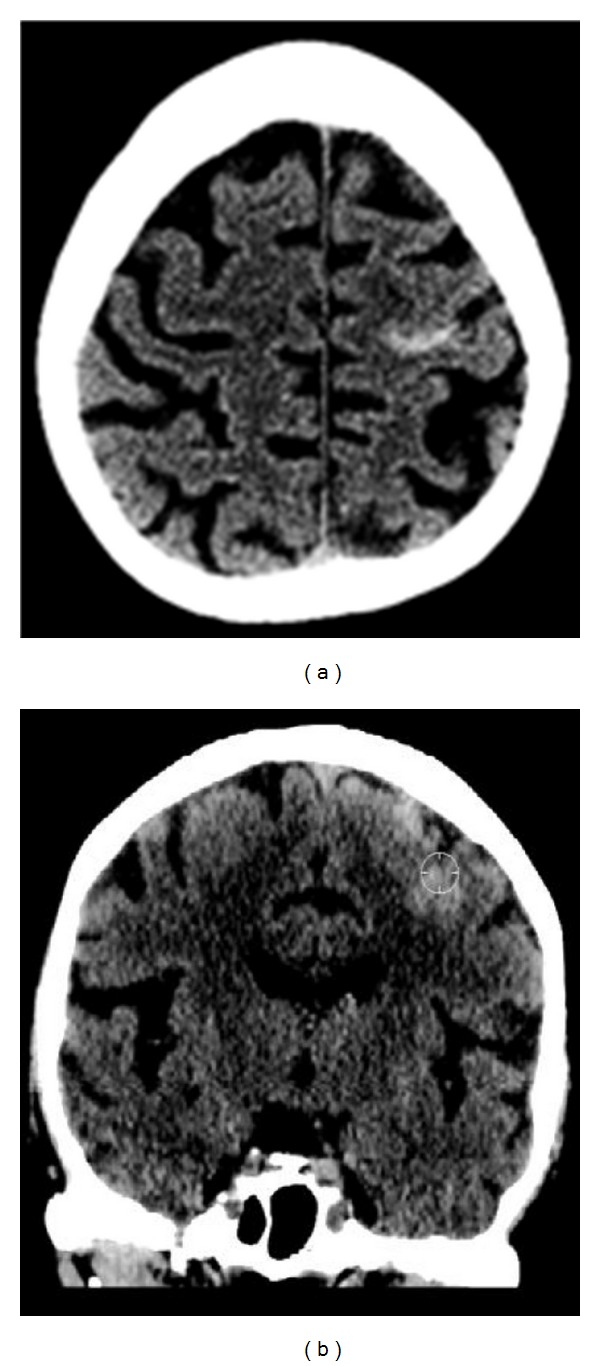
Unenhanced CT images of the brain. Axial and coronal CT images ((a) and (b)) of the brain in an 84-year-old normotensive female without history of trauma demonstrate a linear area of increased attenuation within the left frontal convexity, consistent with an isolated central sulcus hemorrhage.
